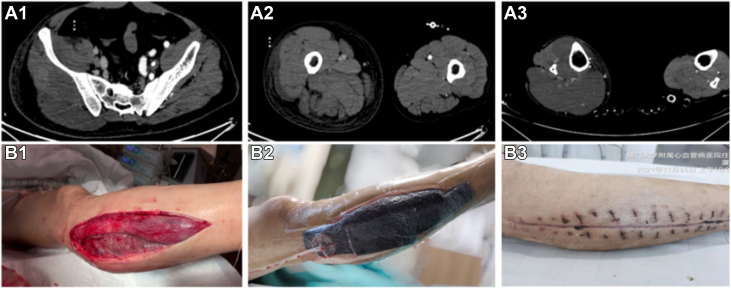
Acute Compartment Syndrome of the Right Lower Limb and Fasciotomy Decompression (A1-A3) Computed tomography showing the swelling of the right lower limb, ipsilateral thigh (A1), buttocks (A2), and waist (A3). (B1-B3) Injury and recovery of the right lower limb. Emergency fasciotomy decompression showed the anterior and lateral calf muscles displayed severely compromised blood supply (B1), vacuum-assisted wound closure (B2), and sutured wound without infection (B3).
